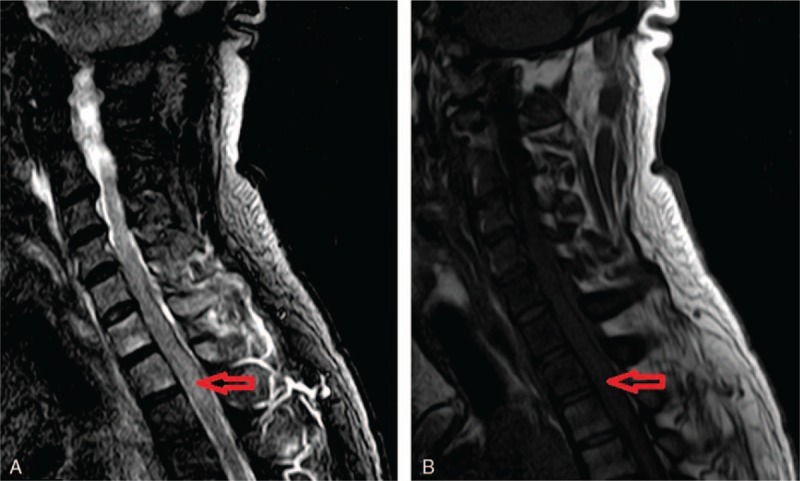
MRI of the cervical spinal cord. A, The T2-weighted images showing uneven increased signal intensity at C4 to T1 level (red arrow). B, The T1-weighted images showing obvious spinal cord thickening at C4 to T1 level (red arrow). MRI = magnetic resonance imaging.
